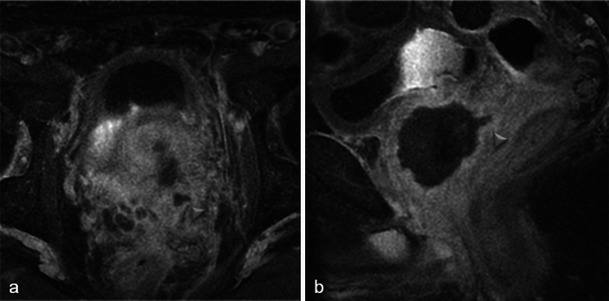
Seminal vesicle. Left photodynamic therapy in a 56-year-old patient. Day-7 post-treatment axial (a) and sagittal (b) T1 FS contrast-enhanced MR images show a small extension of necrosis to the left seminal vesicle (*arrowhead*) by extraprostatic sliding of an optical fibre
